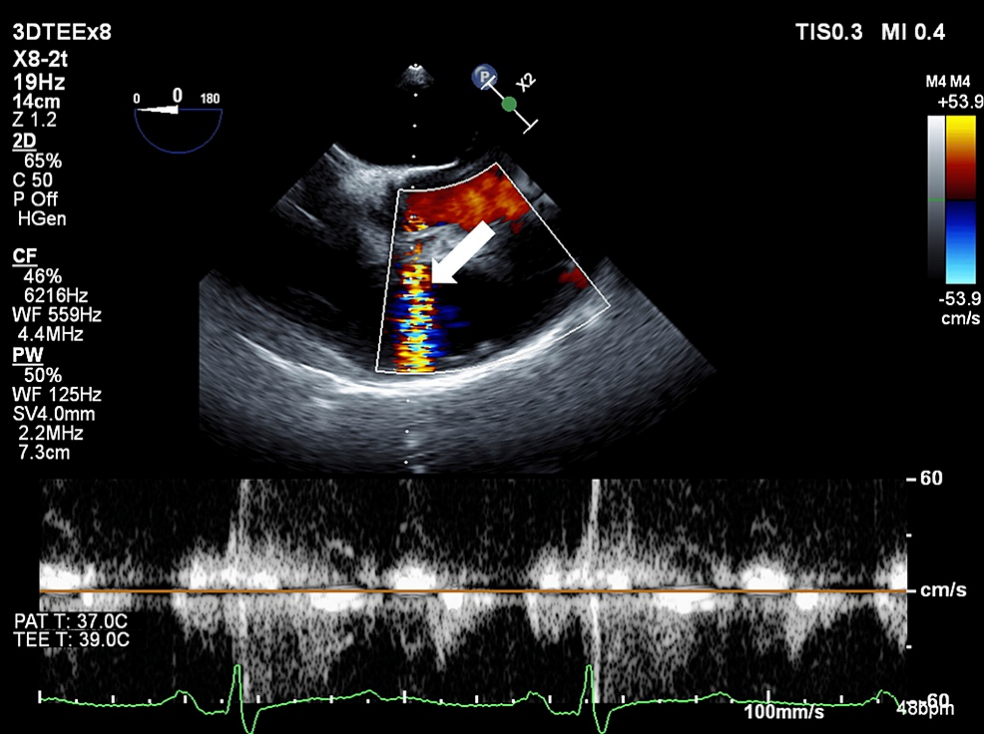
Evaluation of right coronary artery blood flow by pulsed Doppler in a transesophageal echocardiographic image (aortic valve level, short axis view) after induction of anesthesia (arrow: direction of blood flow in the right coronary artery).
